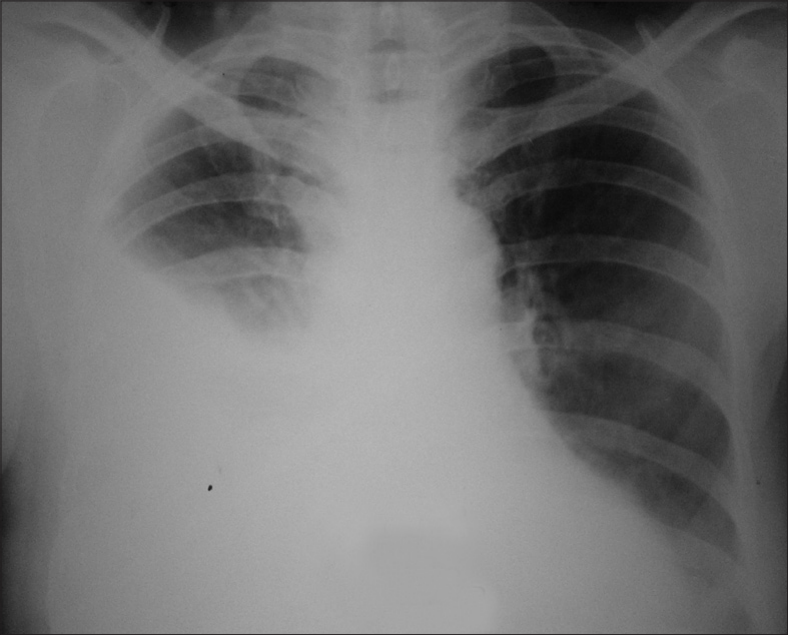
Chest radiograph at the time of presentation
